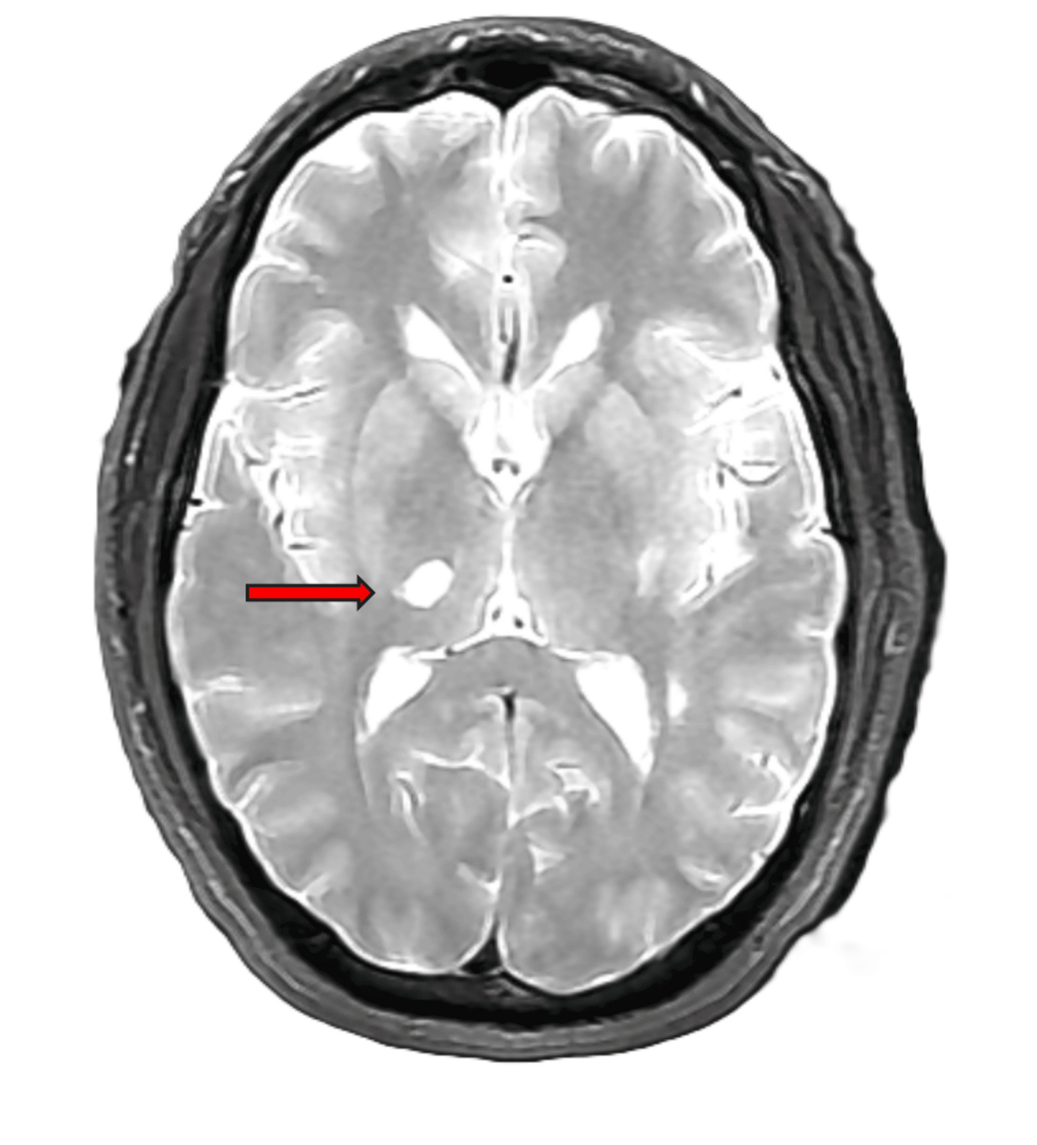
Axial T2 MRI of the brain without contrast
